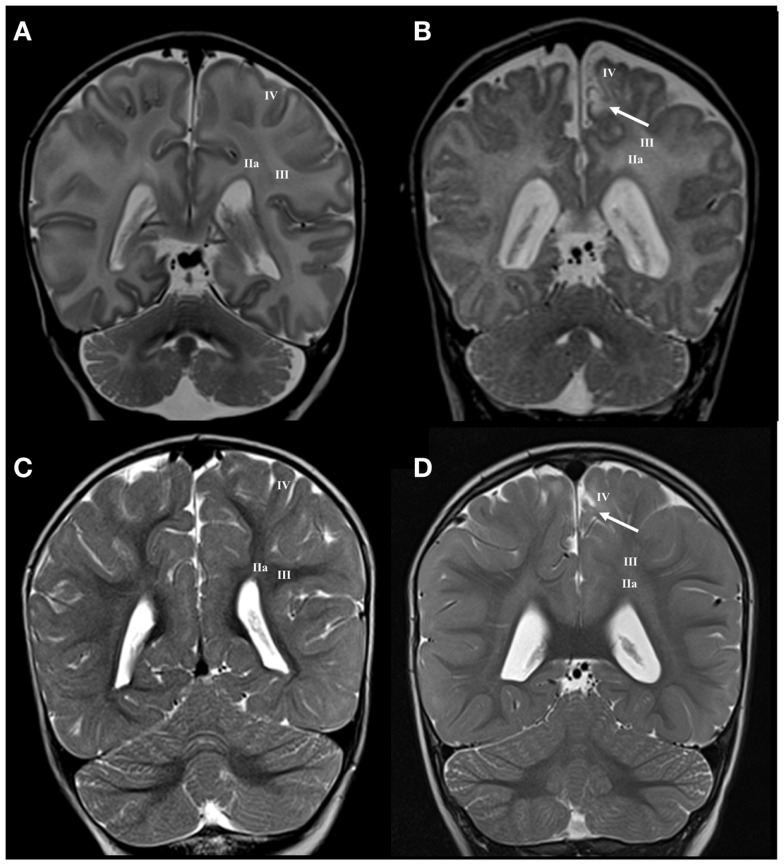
Longitudinal MRI follow-up of perinatal gyral white matter and cerebral cortex lesion on coronal T2 images. Normal findings of gyral white matter and cortex at term age (A) and at the age of 13 months (C). Term born child (BW = 3220 g, SNAPII = 31, SNAPEII = 49, AS = 1/3) with perinatal asphyxia and hyperintensity of centrum semiovale and gyral white matter accompanied with cortical lesion at term equivalent age [arrow in (B)] and with hyper-intensive lesion of gyral white matter and cortex at the age of 13 months [arrow in (D)]. Numbers I–IV represent segments of white matter as previously described.
